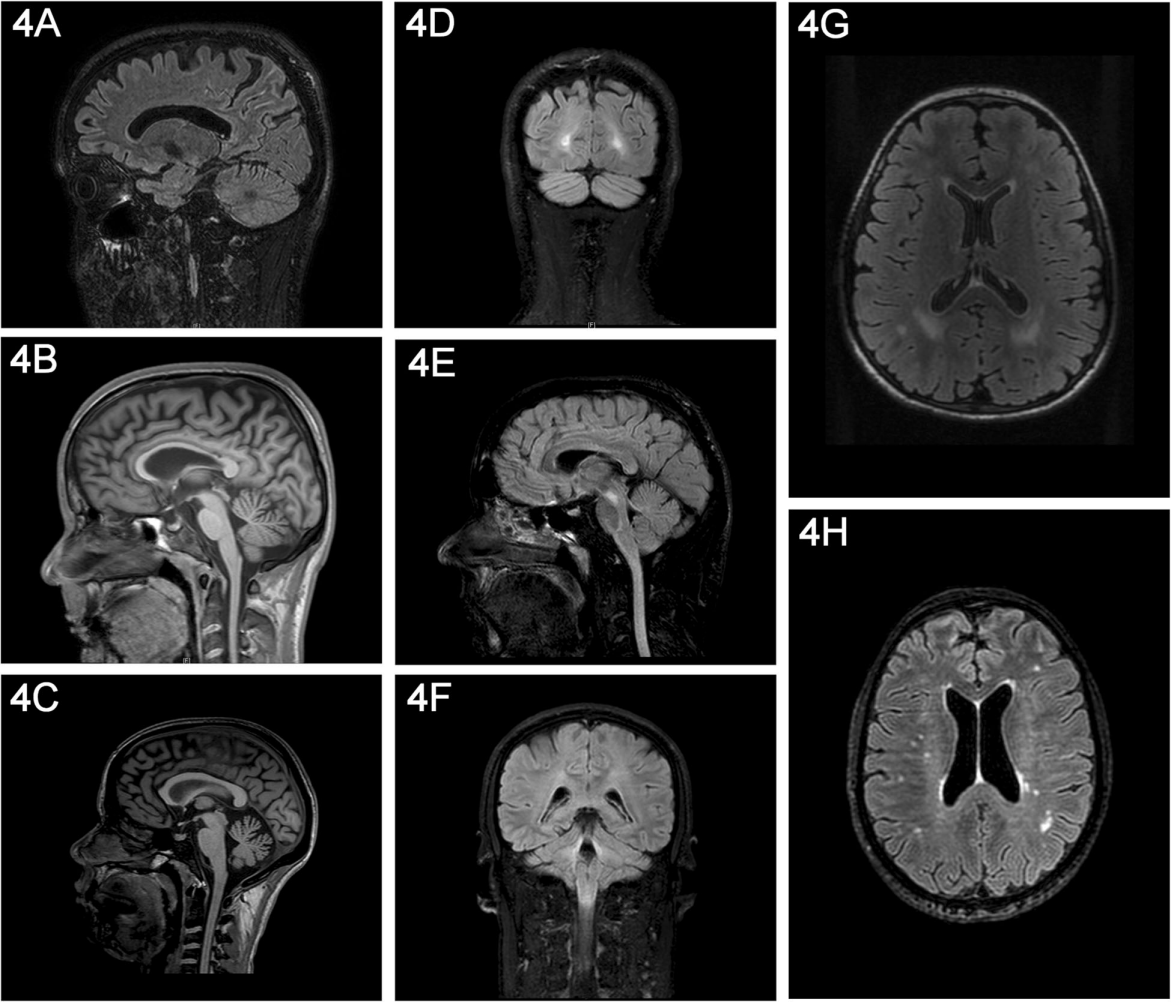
Brain MRI in cystinosis patients (a: parietal atrophy with meningeal hypersignal surrounding the precuneus; b: corpus callosum atrophy; c: vermis atrophy; d: occipital FLAIR hypersignals; e: ponto-mesencephalic FLAIR hypersignals; f: FLAIR hypersignals located around the 4th ventricle including cerebellar peduncles; g-h: more diffuse FLAIR hypersignals)
